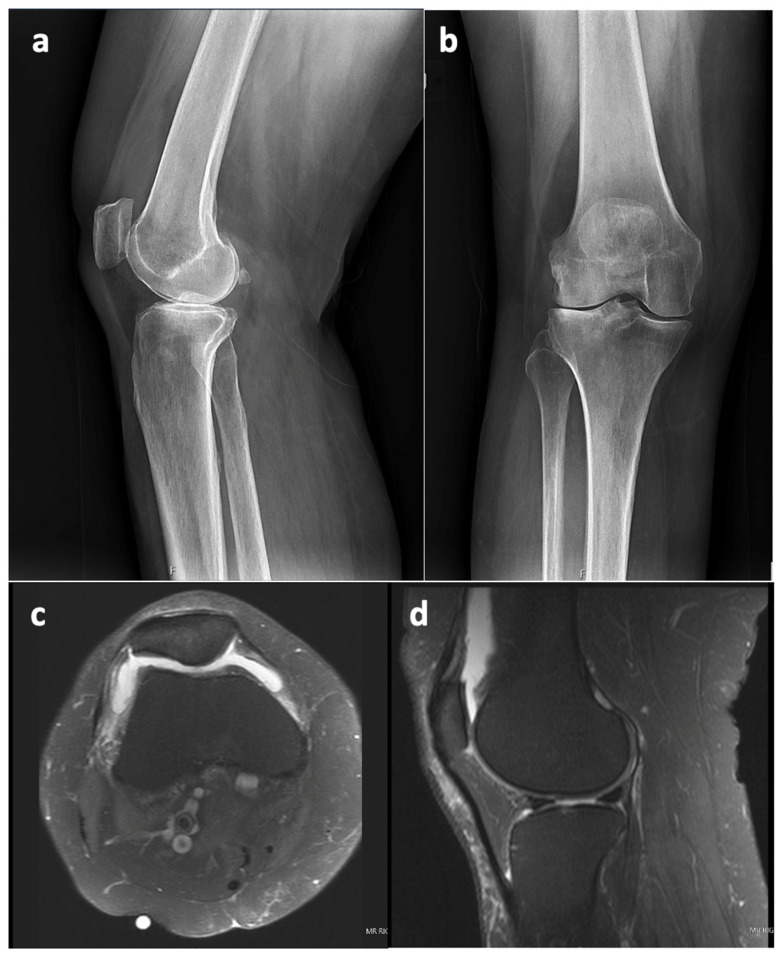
X-ray from 11 June 2018 ((a)—lateral view, (b)—anterior–posterior view) and MRI from 13 March 2017 ((c)—axial view, (d)—sagittal view); right knee shows knee osteoarthritis with no sign of synovial chondromatosis prior to TKA in August 2018.
